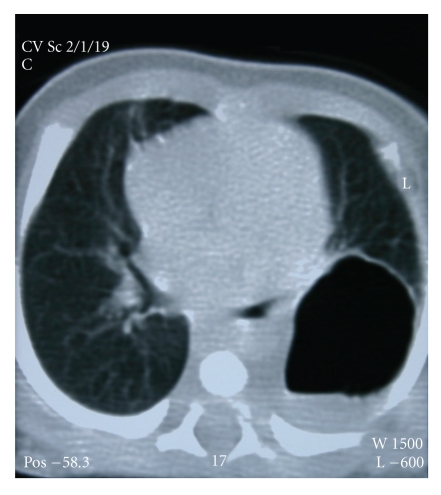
CT imaging of the ballooning of the cyst after birth.
